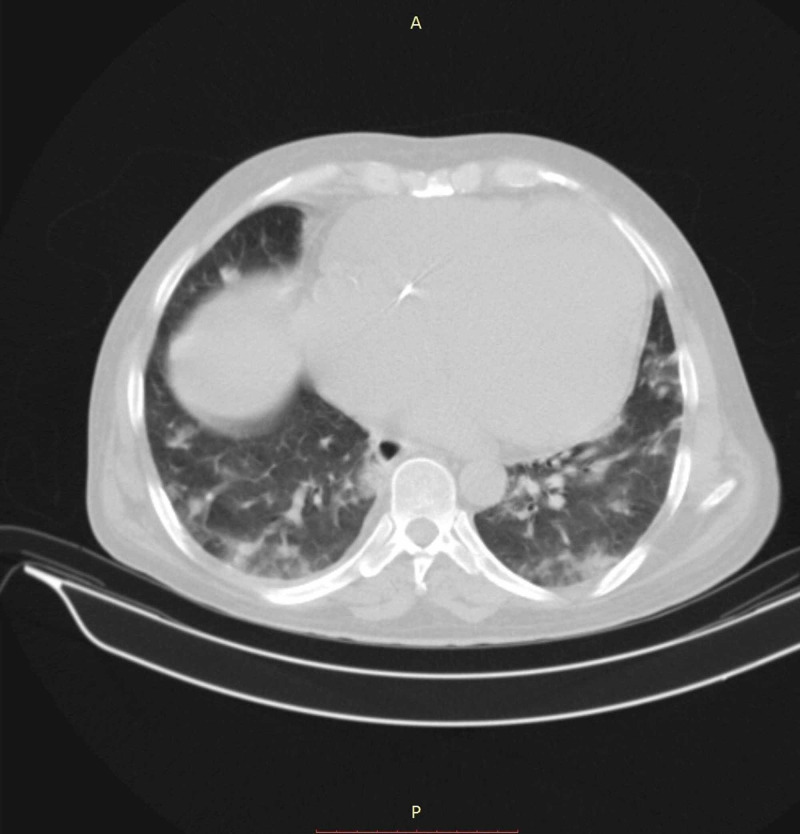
The patient's thoracic CT scan without contrast.
